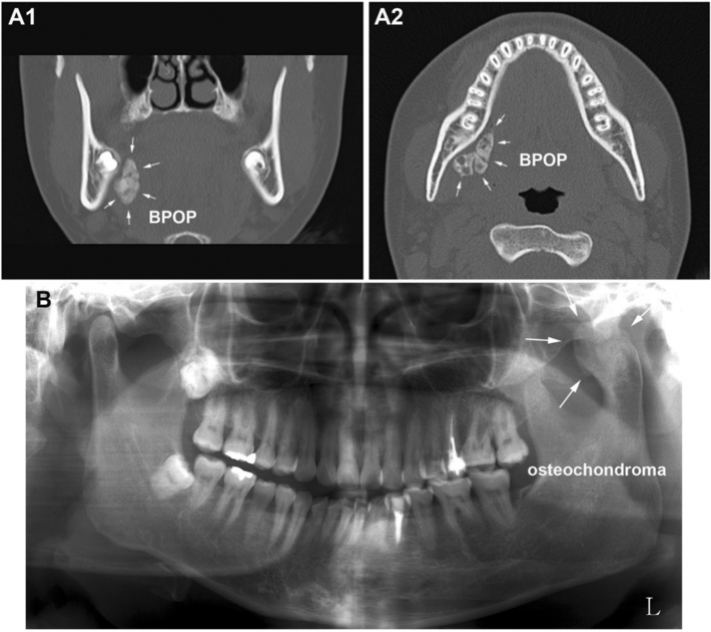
Radiographic views of this study. A Computed tomography of BPOP, an irregularly calcified mass located on the lingual side of the mandibular body without cortical attachment (*arrows*). A1 Frontal plane. A2 Horizontal plane. B Panoramic view of osteochondroma, enlarged left condylar head (*arrows*)
